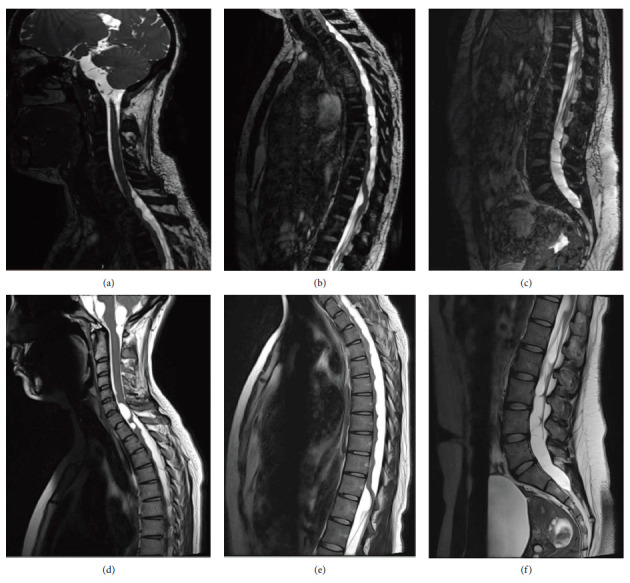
2014 CISS (a)–(c) and 2020 T2-weighted MRI (d)–(f). In (a) and (d), there are cysts in the posterior and anterior rachymedullar subarachnoid space; at the thoracic level in (b) and (e), there is a cystic conglomerate that compresses and displaces the spinal cord surface; in (c) and (f), multiple cysts displacing the nerve roots are observed.
